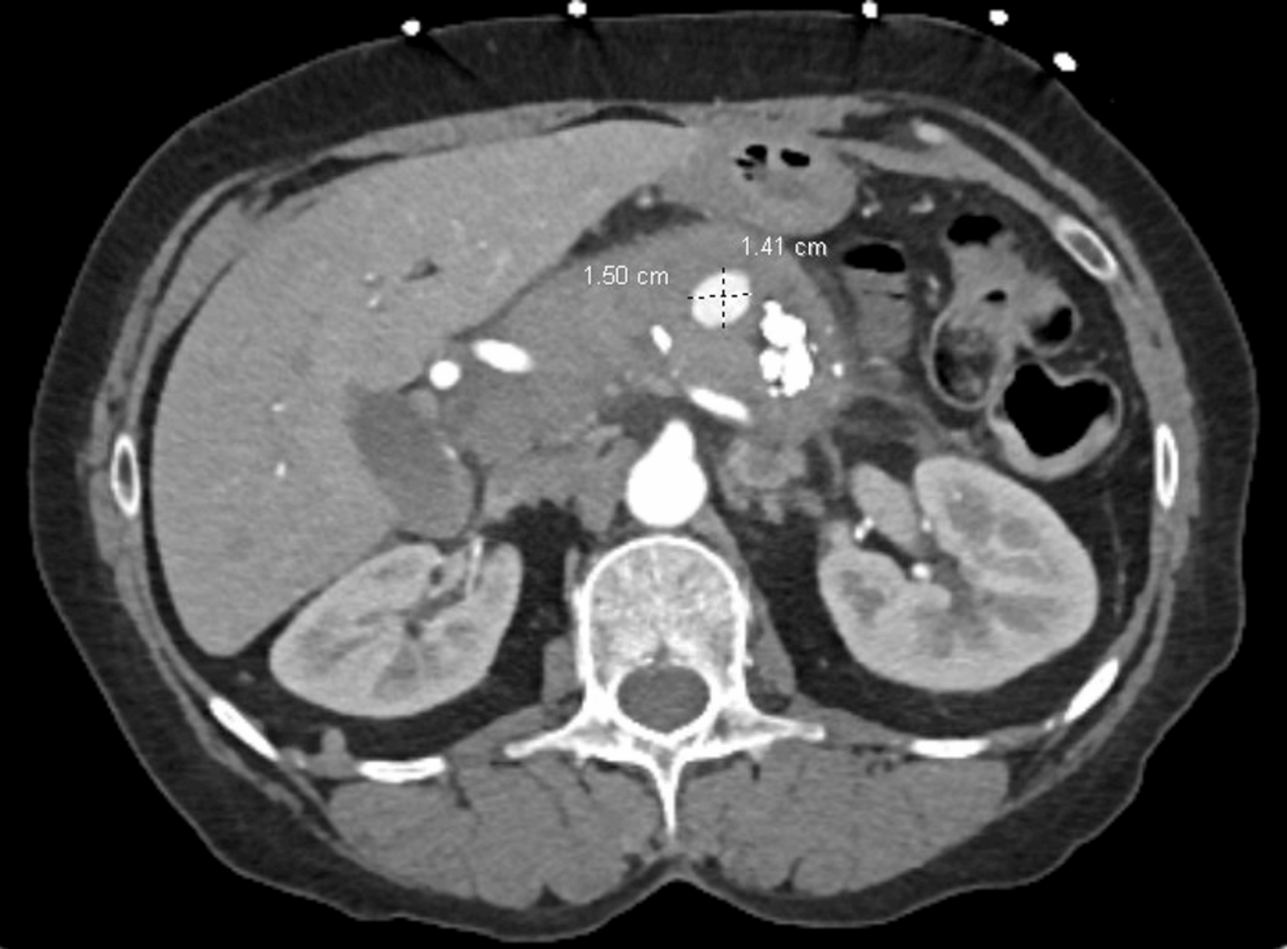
Left gastric artery pseudoaneurysm. CT angiogram of the abdomen and pelvis with intravenous contrast (axial view). Noted to be measuring 1.5 × 1.4 cm.
